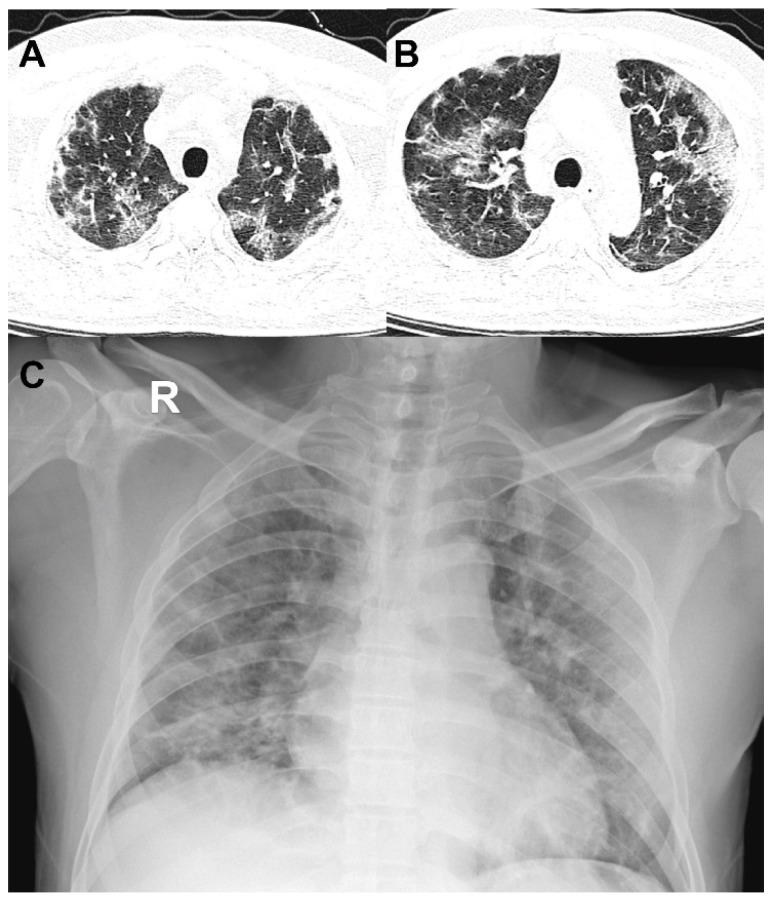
52-year-old man with coronavirus disease 2019. A-B. Non-contrast CT was performed on day of admission. C. Chest X-ray was performed on day of admission. Patient developed acute respiratory failure at the second day of hospitalization. Chest CT images showed large areas of bilateral consolidation and ground-glass opacities in upper lungs and lesions showed peripheral distribution. Consist with CT, chest X-ray also showed patchy consolidation in bilateral lung periphery.
